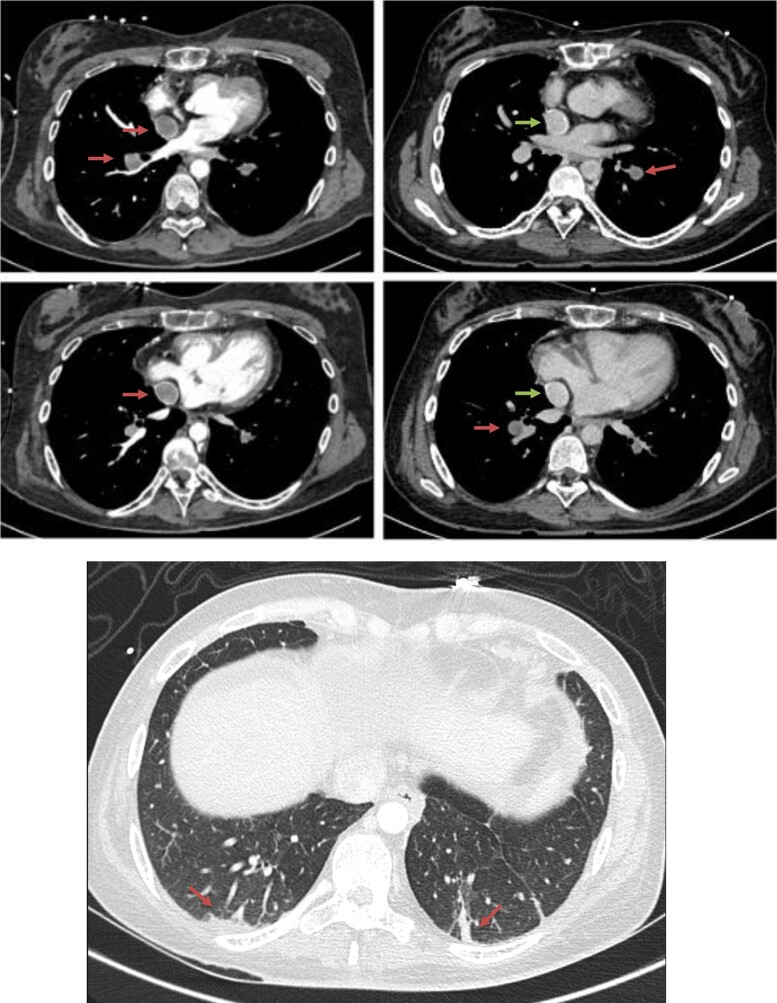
Comparison between chest computed tomography performed without (left) and with (right) Fontan protocol. Left images show pulmonary thromboembolism and Fontan thrombosis (arrows) while right images, taken with a very delayed acquisition, evidence permeability of the Fontan circuit (upper arrows) with persistance of pulmonary thromboembolism (lower arrows). The lower image (lung window) shows bilateral pulmonary infarcts (arrows).
